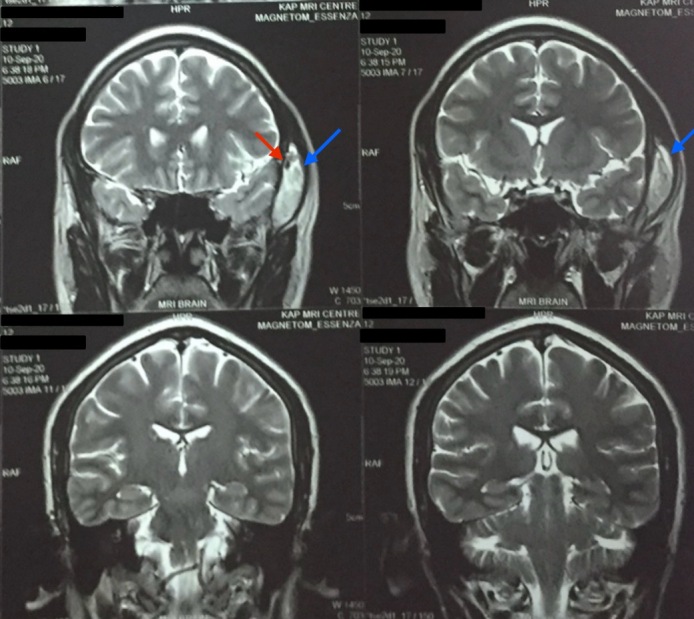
A 4.3 x 3.3 x 1.3 cm heterogenous lesion in the temporalis muscle (indicated with blue arrows), hyperintense on T2 weighted brain and neck MRI (coronal view) with foci of calcification (indicated with red arrow).
